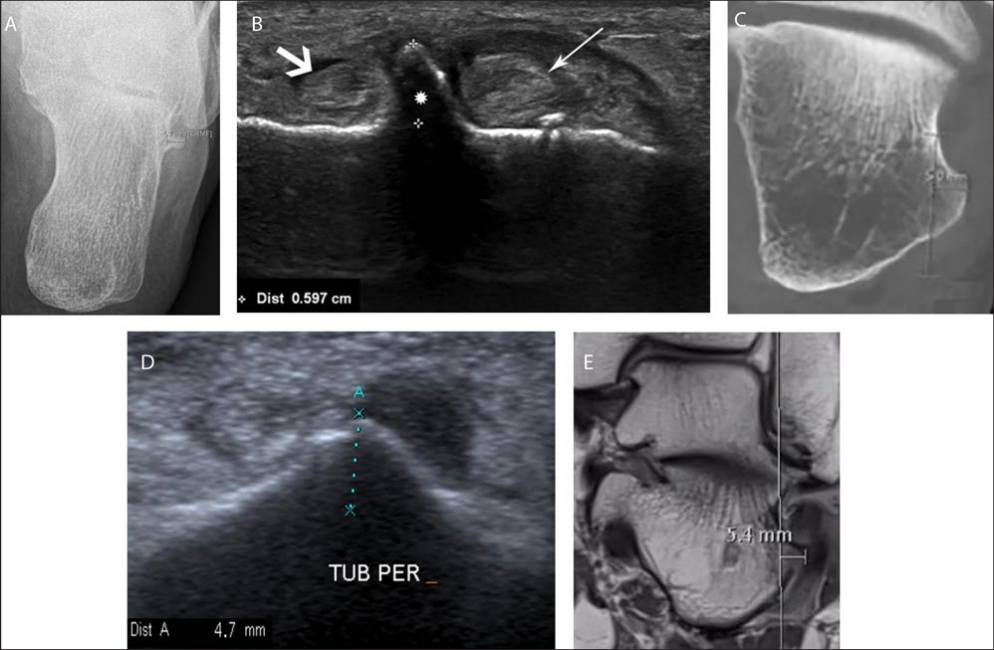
Examples of measurement of the width of the TP on different imaging methods. Axial CR (A) shows a hypertrophic TP with a maximum width of 5.7 mm measured between the base and the top of the tuberculum. Oblique coronal US (B) confirmed the presence of a hypertrophic TP (white asterisks) with a measured width located between the course of the peroneus longus (thin white arrow) and brevis (thick white arrow). Coronal CBCT (C) reveals a hypertrophic tuberculum peroneum in another patient with a width of 5 mm. This measurement correlates well with the width measured on the oblique coronal ultrasound in the same patient (D). Coronal PD MR image demonstrating the width measurement of the hypertrophic tuberculum peroneum (E).
